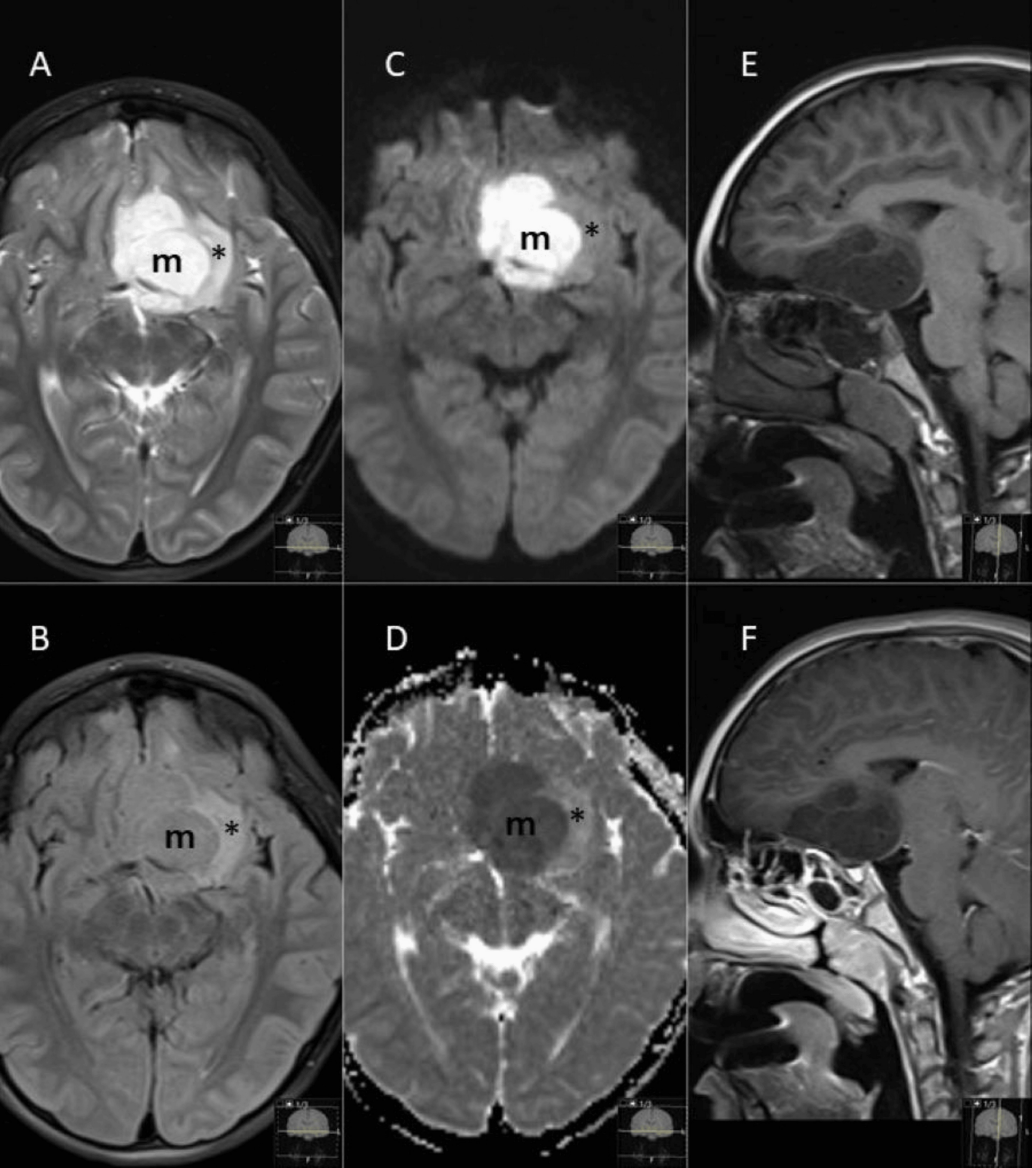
MRI of the brain revealed a 35 x 41 x 38-mm intra-axial mass (m) at the left inferior frontal lobe situated atop the planum sphenoidale, encasing the left anterior cerebral artery (ACA) and deviating the optic chiasm and left optic tract. The mass was hyperintense on T2 (A) and isointense on T2/FLAIR (B). Diffusion-weighted imaging (DWI) (C) showed intense proton diffusion restriction with a low apparent diffusion coefficient (ADC) (D). The mass was hypointense on T1 (E) and showed no enhancement after gadolinium on post-contrast T1 (F). Minimal perilesional vasogenic edema can be seen at the periphery (*). T2/FLAIR: T2-weighted fluid-attenuated inversion recovery.
